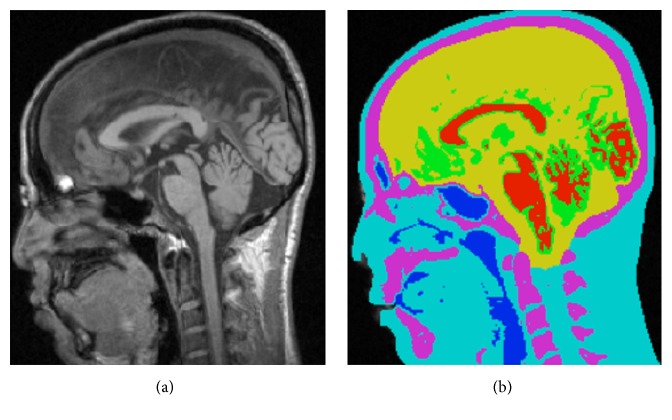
MRI-to-CT registration. (a) Transformed MRI. (b) Fusion of MRI and CT segmentation.
